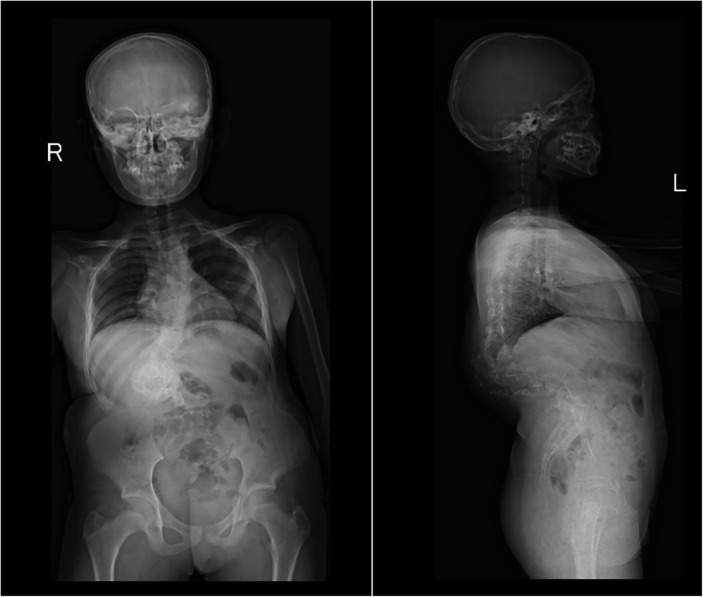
Preoperative full-length spine radiographs (anteroposterior and lateral views) showing S-shaped scoliosis.
